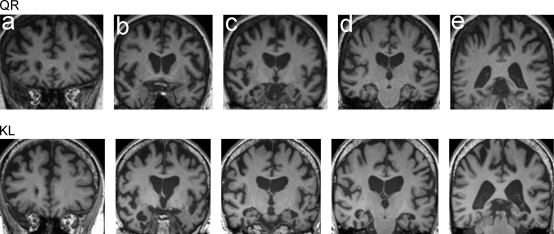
Representative T1-weighted coronal brain MRI sections from each patient (the right hemisphere is shown on the left side of each image). Sections have been selected to show the following regions of potential relevance to voice processing deficits: a, frontal lobes; b, temporal poles; c, anterior temporal lobes; d, mid-temporal lobes including Heschl's gyri; e, temporo-parietal junction. Focal cerebral atrophy is shown in both patients: in QR, bilateral fronto-temporal atrophy accentuated in the right anterior temporal lobe and extending posteriorly and including the superior temporal sulcus; and in KL, bilateral predominantly anterior temporal lobe atrophy, more marked on the right side and in the inferior temporal cortices including the fusiform gyrus.
